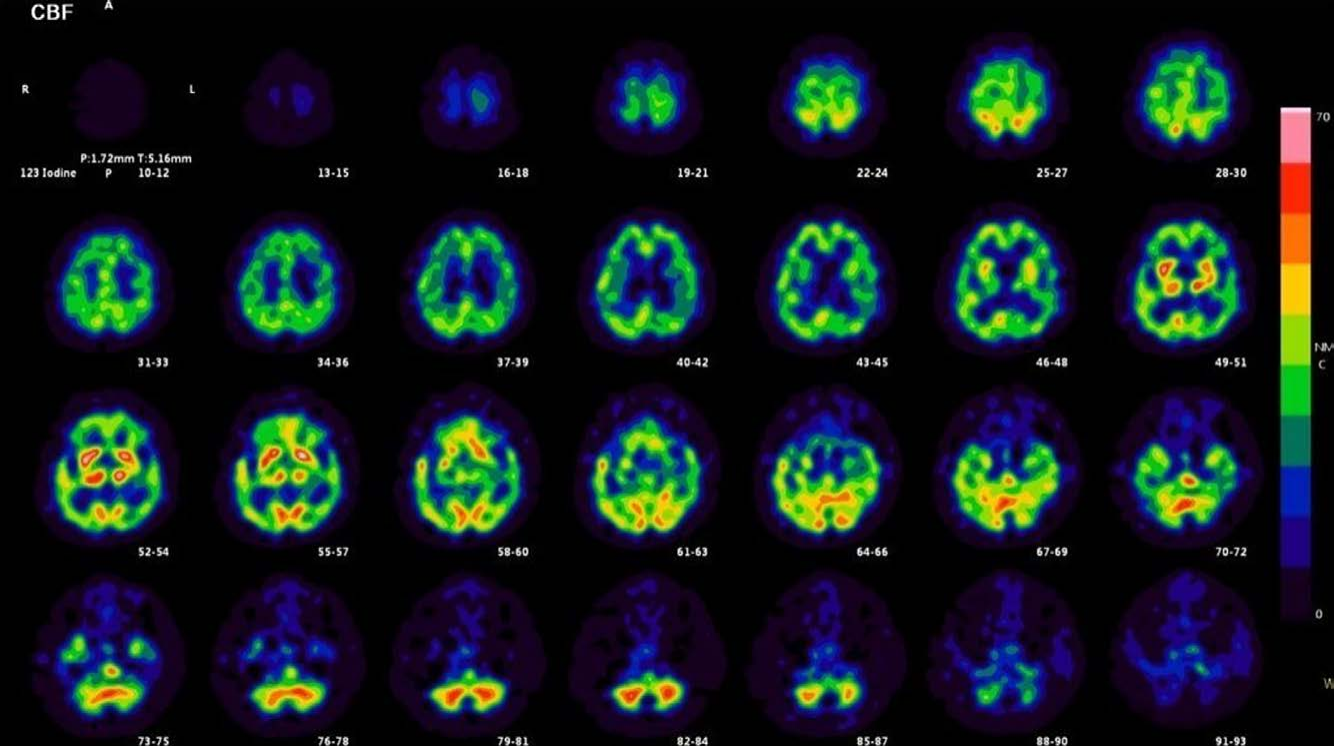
Brain perfusion single-photon emission computed tomography (^123^I-IMP). Low blood flow was observed in the posterior parietal lobe as well as in the parietal and, frontal cortices, suggesting diffuse function impairment of the brain due to encephalopathy.
